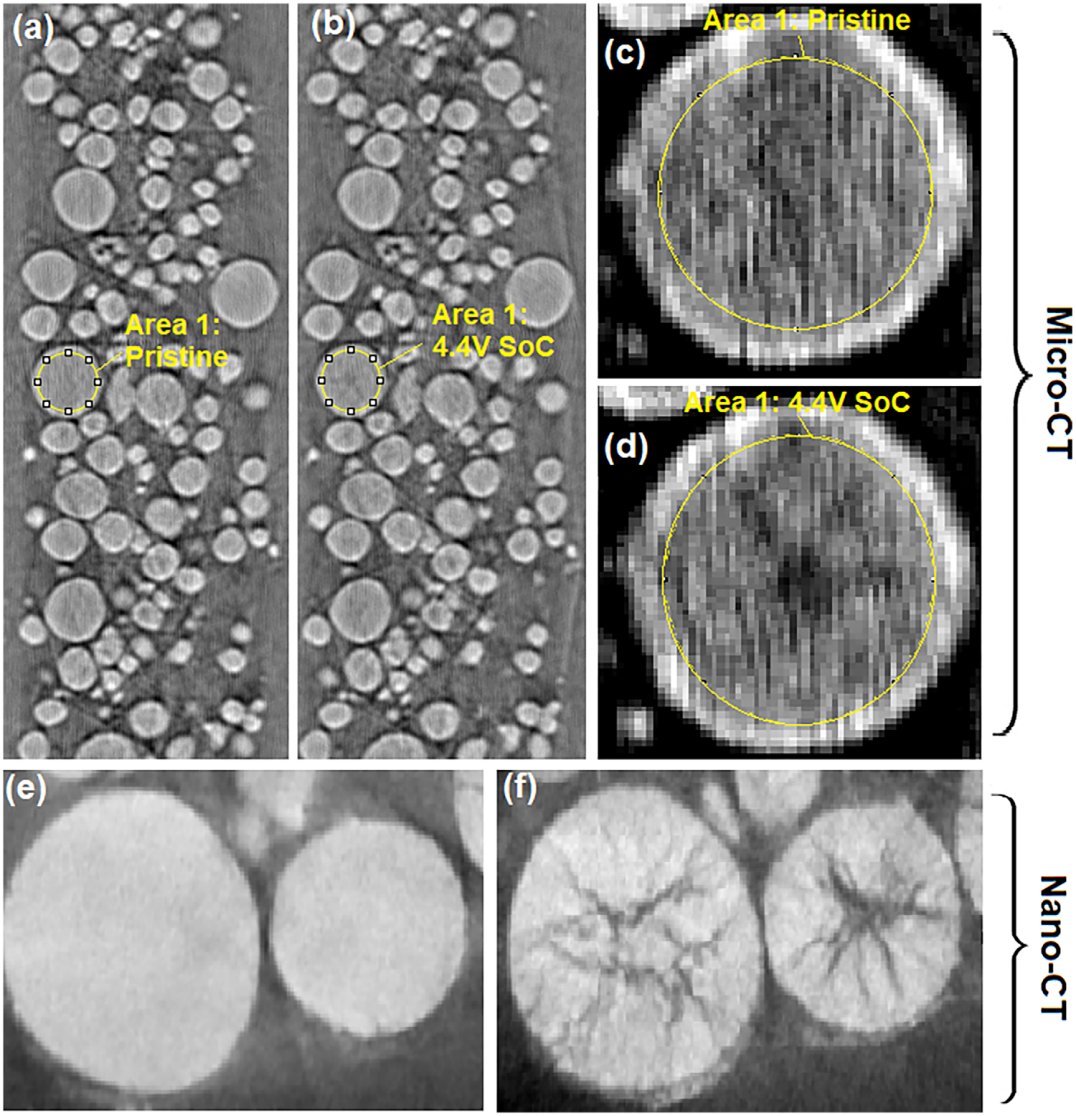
The partial volume effect of sub‐resolution cracks in Micro‐CT images of NMC particles. a) Micro‐CT slice showing pristine NMC particles with Area 1 highlighted, b) the same micro‐CT ROI at 4.4V SoC with Area 1 highlighted. c) A high contrast & magnified micro‐CT view of area 1 when pristine. The mean grey‐level in area 1 is 148.0. d) A high contrast & magnified micro‐CT view of area 1 at 4.4V SoC. The mean grey‐level in area 1 is now 147.1. e) Nano‐CT of a pristine NMC particle, f) Nano‐CT of a NMC particle at 4.5V SoC. Nano‐CT courtesy of Parks et al.,^[^
